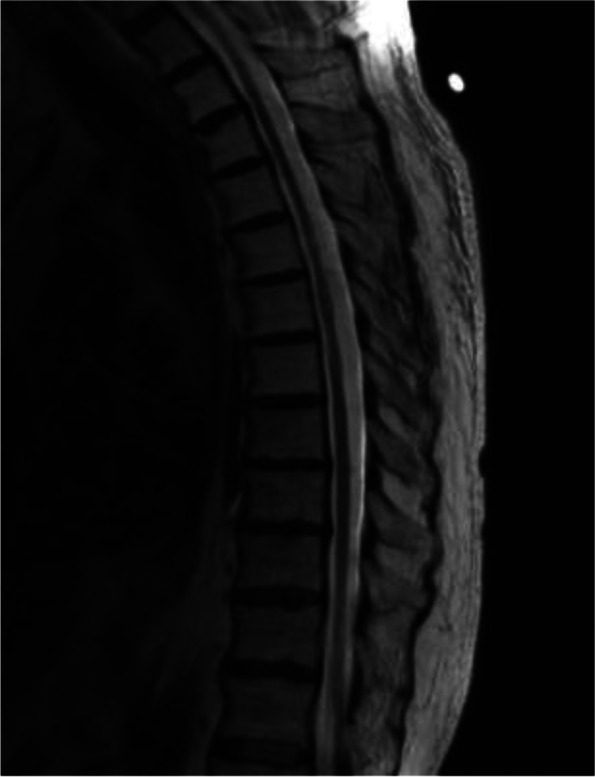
MRI thoracic spine: Sagittal T2: After 6 cycles of cyclophosphamide treatment: resolving of T2 signal. Atrophy of the spinal cord
